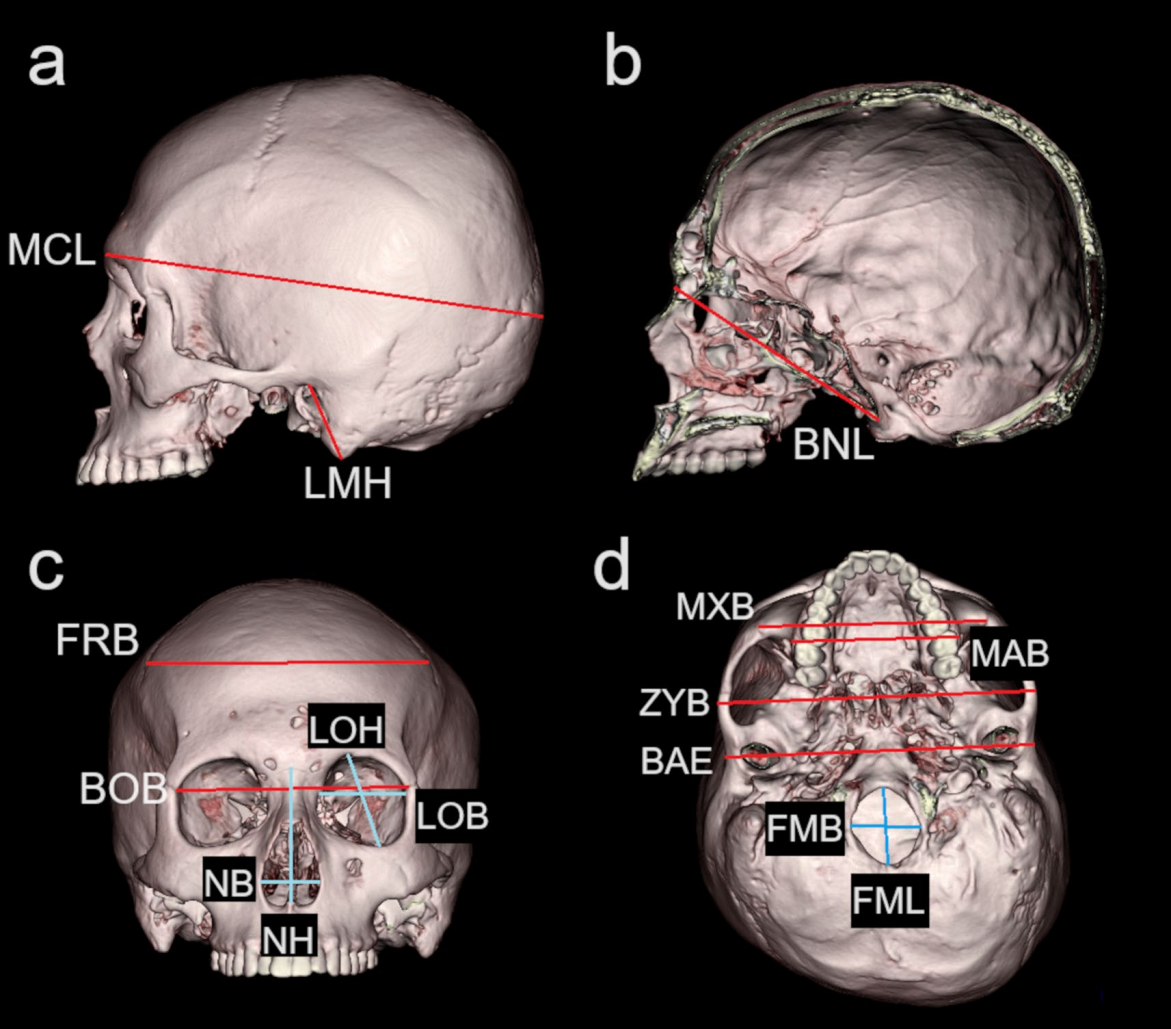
Three-dimensional computed tomography images presenting cranial measurements (see Online Resource 2 for their definitions): (a) maximum cranial length (MCL) and left mastoid height (LMH); (b) basion–nasion length (BNL); (c) frontal breadth (FRB), biorbital breadth (BOB), left orbit height (LOH), left orbit breadth (LOB), nasal height (NH), and nasal breadth (NB); and (d) bimaxillary breadth (MXB), maxillo-alveolar breadth (MAB), bizygomatic breadth (ZYB), biauricular breadth (BAE), foramen magnum length (FML), and foramen magnum breadth (FMB). Right mastoid height (RMH), right orbit height (ROH), and right orbit breadth (ROB) are not shown because they are left symmetrical
